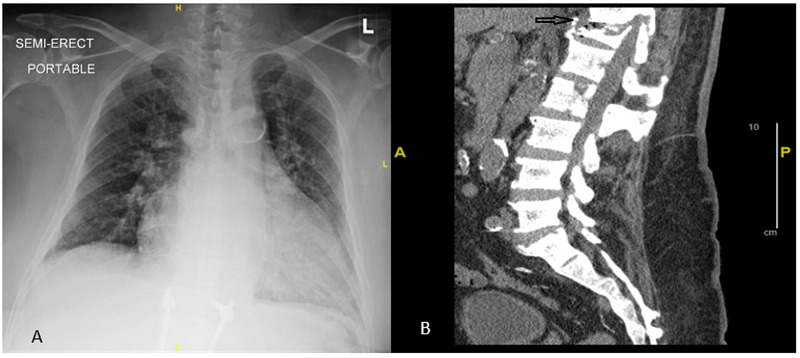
Chest X-ray and lumbar spine CT scan of the patient A: the chest X-ray revealed no active chest disease. B: the lumbar spine CT scan without contrast revealed chronic erosion and sclerosis at the T11-12 with increased loss of height, erosion at the T12, and paraspinal soft tissue thickening, raising concern for acute on chronic osteomyelitis CT: computed tomography
